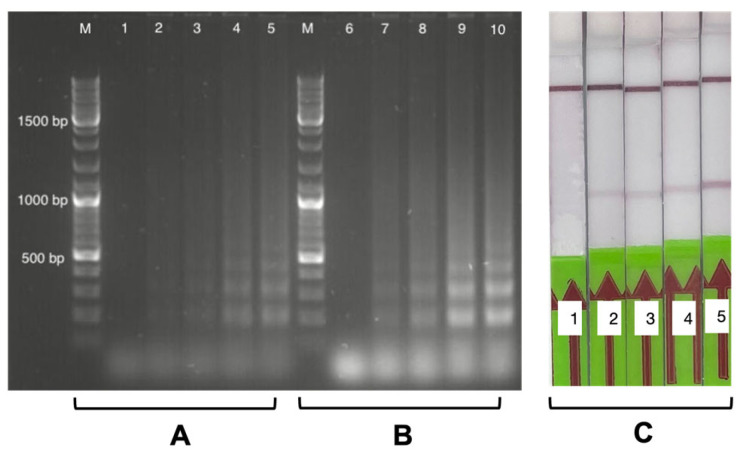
RT-LAMP for SARS-CoV-2 positive swab samples (CT value 18.55) in a 20 min of processing time. RT-LAMP results were read out on 1.5% agarose gel, and the gel image shows five RT-LAMP amplicon results before and after biotinylated-probe hybridization: one NTC and four quadruplets of positive sample with CT 18.55. (A) RT-LAMP results before biotinylated-probe hybridization: firstly, the M-100 bp DNA marker; then, NTC in lane 1; lanes 2 to 5 are 4 positive swab sample amplicons. (B) RT-LAMP results after biotinylated-probe hybridization: firstly, the M-100 bp DNA marker; lane 6 is NTC with all biotinylated-probes ABC; lane 7 is positive RT-LAMP amplicon with probe A; lane 8 is positive RT-LAMP amplicon with probe B; lane 9 is positive RT-LAMP amplicon with probe C; and lane 10 is positive RT-LAMP amplicon with all biotinylated-probes ABC. (C) LFA readout for the bi-labelled RT-LAMP amplicon: LFA 1 NTC with biotinylated-probes ABC; LFA 2 is positive RT-LAMP amplicon with probe A; LFA 3 is positive RT-LAMP amplicon with probe B; LFA 4 is positive RT-LAMP amplicon with probe C; and LFA 5 is positive RT-LAMP amplicon with all biotinylated-probes ABC.
